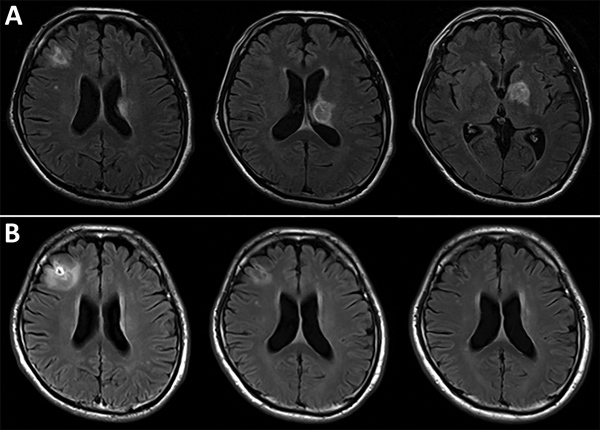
Serial brain magnetic resonance imaging results for a 57-year-old man with *Toxoplasma gondii* encephalitis, Tokyo, Japan. A) All 3 lesions were evident when the patient first sought care. B) Chronologic changes are shown of the lesion in the right frontal lobe in response to antitoxoplasmic therapy after 1 (left), 3 (center), and 12 (right) months.
